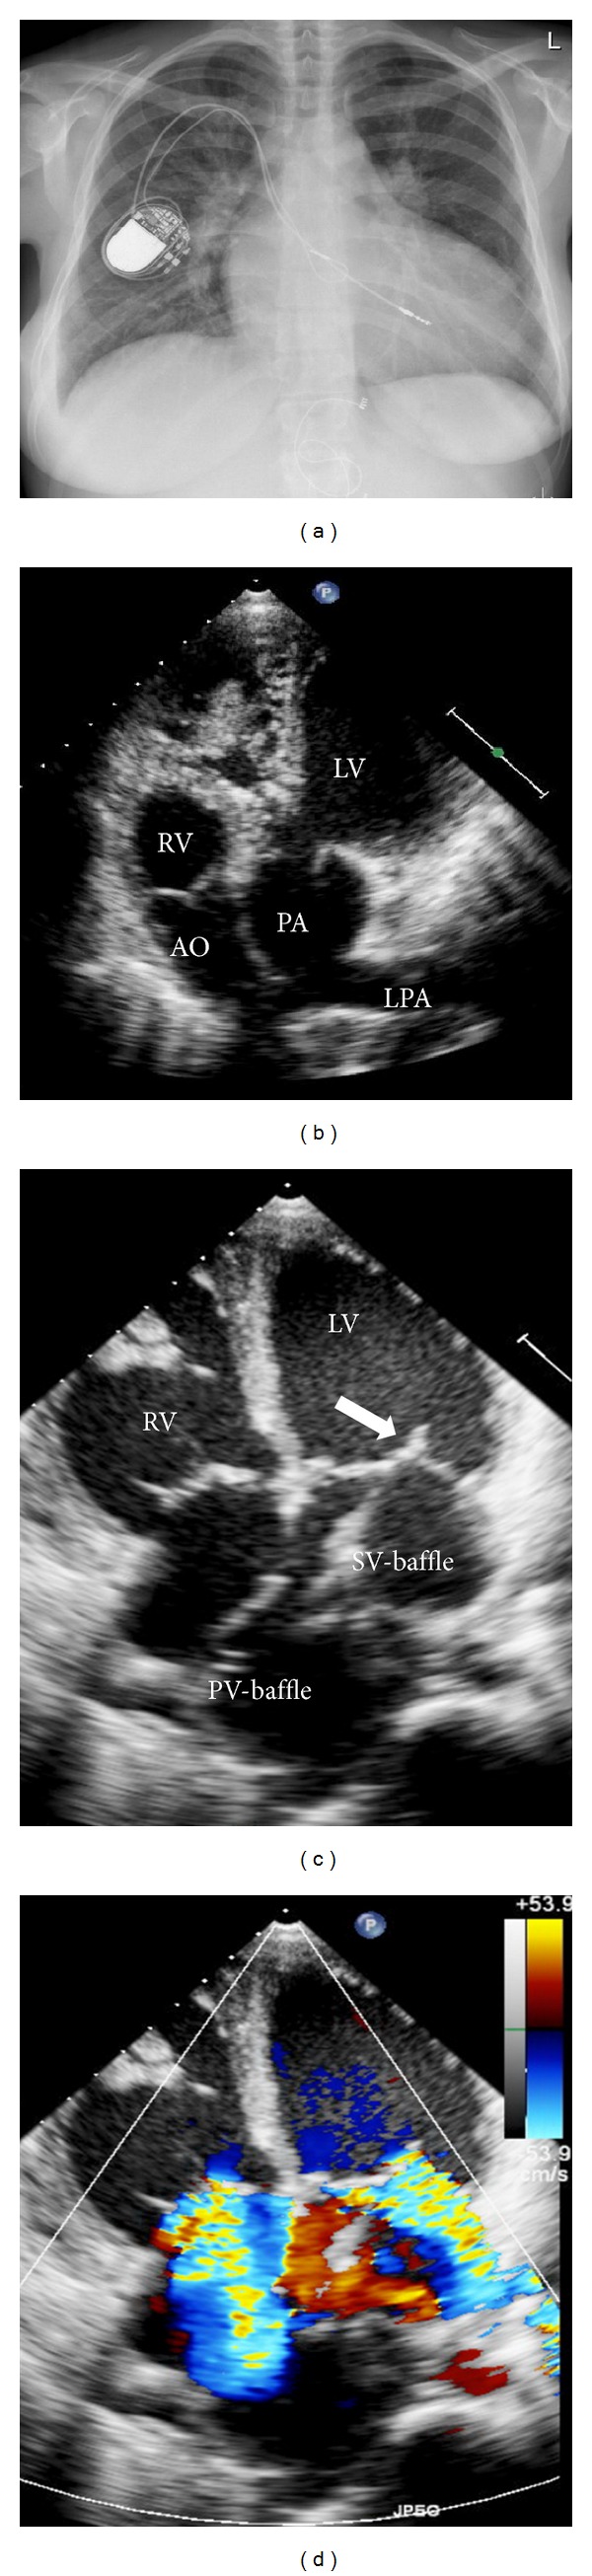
32-year-old patient with severe biventricular dysfunction after atrial switch operation for complete transposition of the great arteries with pacemaker leads in the subpulmonic left ventricle and the systemic venous baffle. (a) Chest X-ray with transvenous pacemaker leads. (b) Apical “5-chamber”-view showing the aorta arising from the morphologic right and the pulmonary artery from the subaortic left ventricle (RV: right ventricle, LV: left ventricle, AO: aorta, PA: main pulmonary artery, LPA: left pulmonary artery). (c) Apical 4-chamber view showing the pulmonary venous and the systemic venous baffles, redirecting venous blood. The arrow points to the pacemaker lead in the subpulmonic left ventricle. (d) Apical 4-chamber view with colour Doppler demonstrating severe (subpulmonic) mitral and severe (systemic) tricuspid regurgitation.
